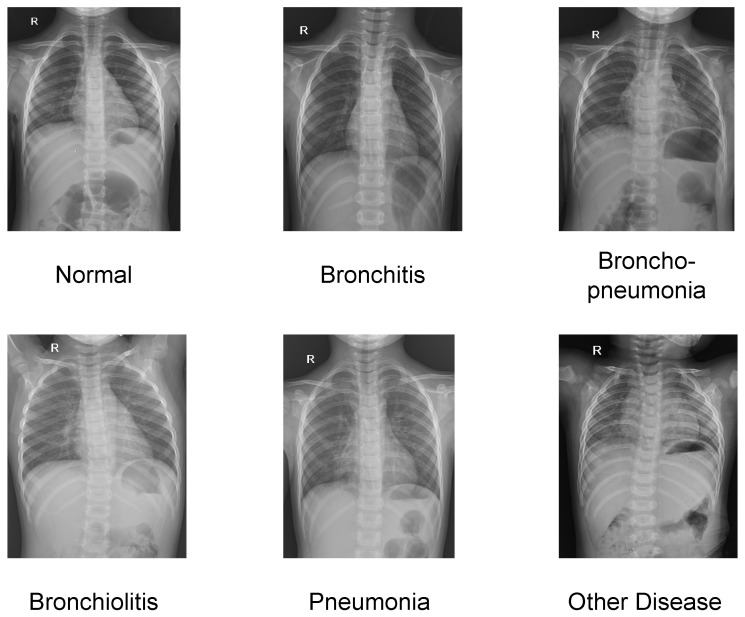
Representative pediatric chest X-ray samples from the VinDr-PCXR dataset, covering six diagnostic categories.
